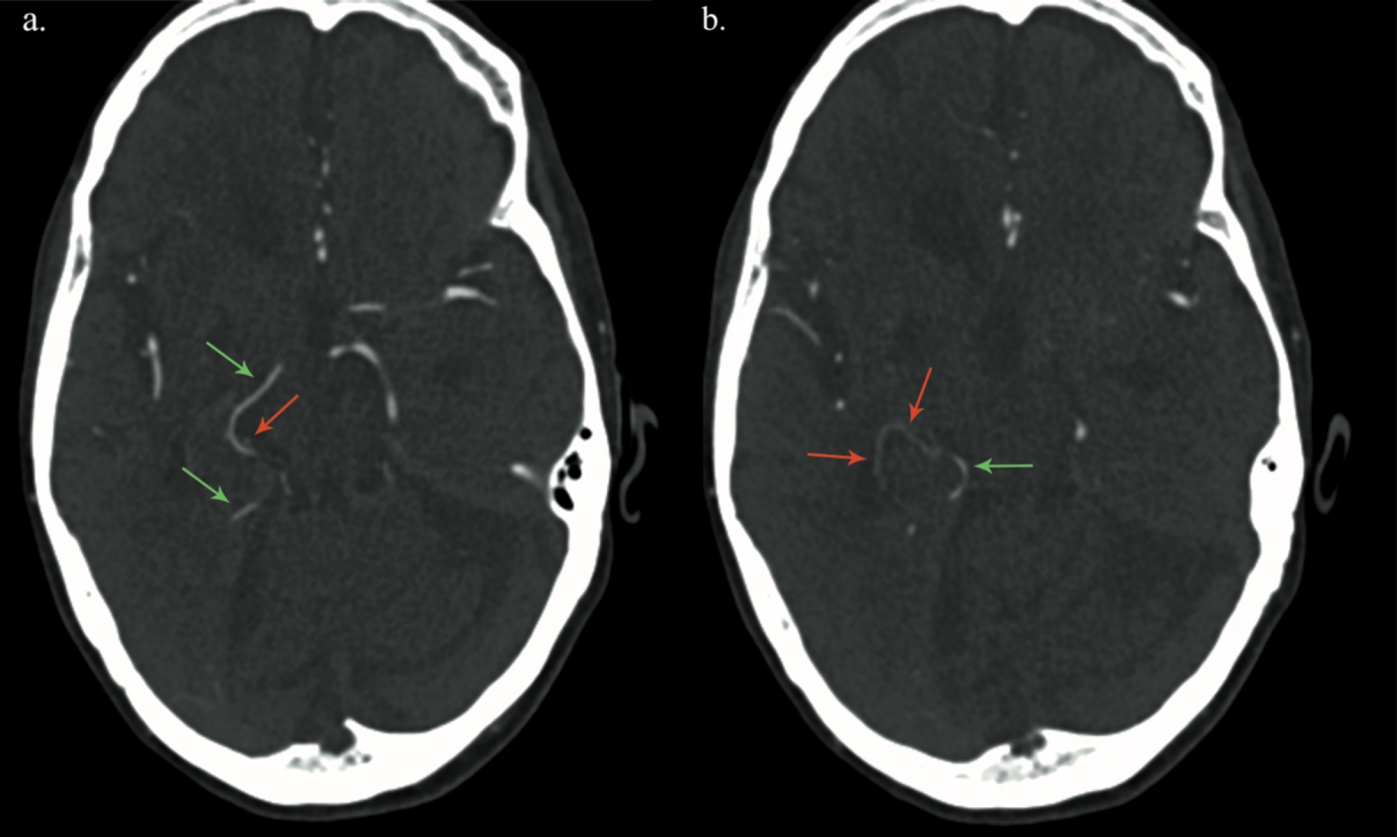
Axial images from CT angiogram of the head through the level of the Foramen of Monroe (a.) and third ventricle (b.) demonstrating the relationship between the fetal right PCA (green arrows) and anomalous AChoA (red arrows) Near the medial aspect of the right side tentorium cerebelli, the anomalous AChoA dives under the P2/3 segment of the fetal right PCA, courses anterolaterally for a short distance, and then ascends to terminate at the choroid plexus in the right lateral ventricle. CT = computed tomography; PCA = posterior cerebral artery; AChoA = anterior choroidal artery
